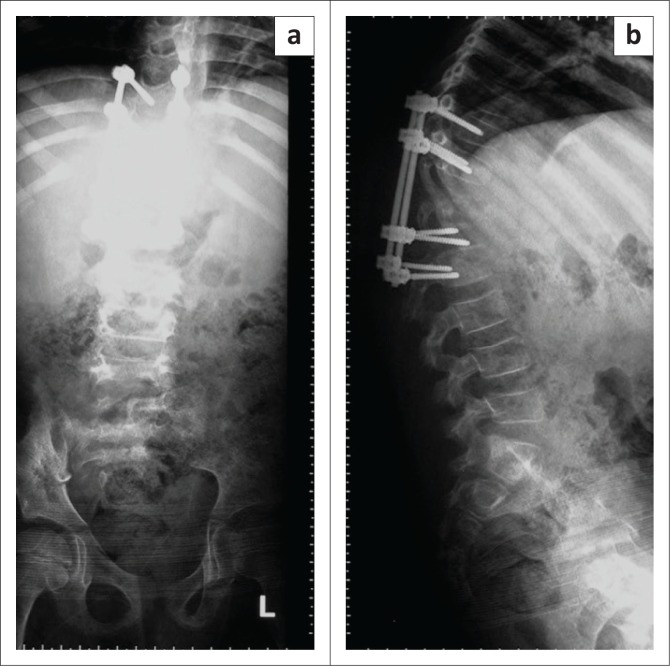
X-rays showing failed implants backing off from the (a) distal part of vertebra (T12/L1) and (b) increased kyphotic deformity of the spine.
